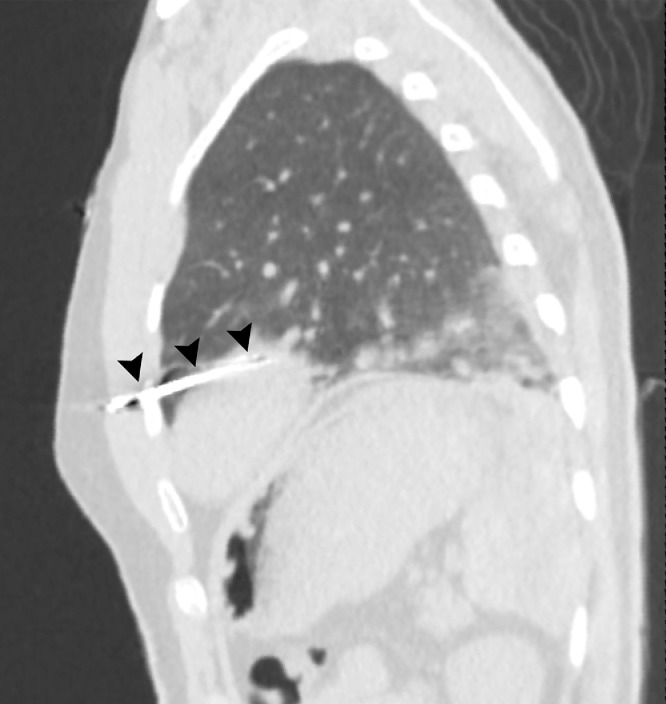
Sagittal view of the chest CT-lung window, the nail is shown (black arrowheads).
